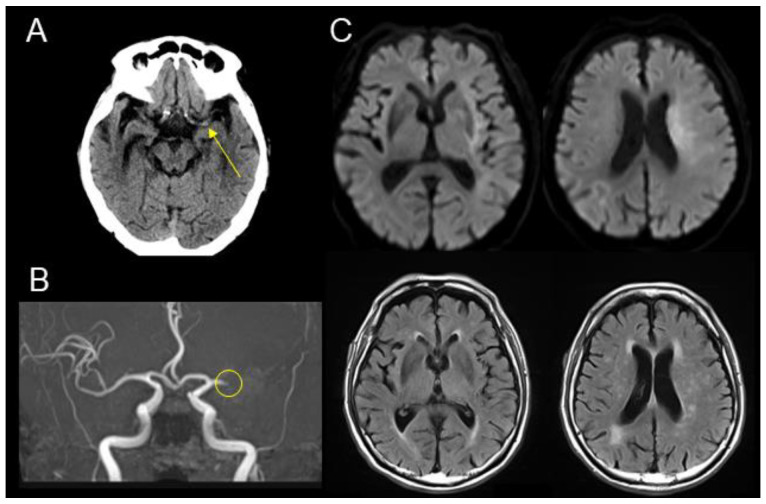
Preprocedural imaging examination upon admission. (A) Brain CT showed a high-density sign of the left middle cerebral artery (arrow). (B) Magnetic resonance angiography revealed a left M1 occlusion (circle). (C) Diffusion-weighted imaging (DWI) (upper raw) showed an ischemic area of the left insular cortex, internal capsule, basal ganglia, corona radiata, and hemispheric subcortical white matter (DWI-ASPECTS of 6 points). No apparent hyperintense signals were identified on fluid-attenuated inversion recovery (FLAIR) images (lower raw), suggestive of DWI–FLAIR mismatch.
